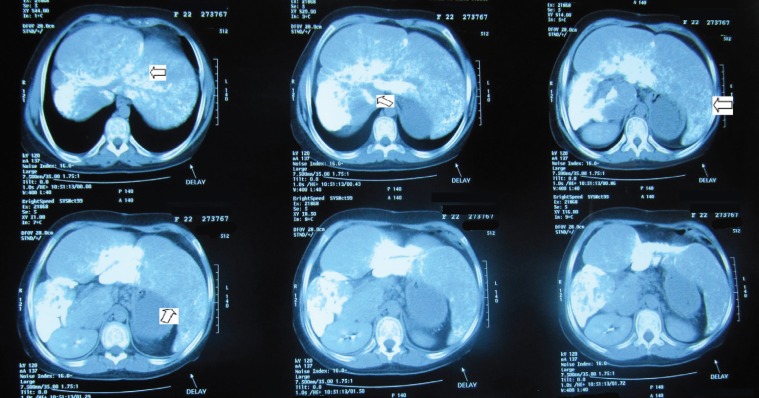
This is abdominal spiral CT-scan with intravenous and oral contrast of the patient, who had beta-thalassemia major and hypoparathyroidism. The arrows point at the hypertrophy of the left and caudate lobes of the liver in addition to severe calcification in the right, left, and caudate lobes
